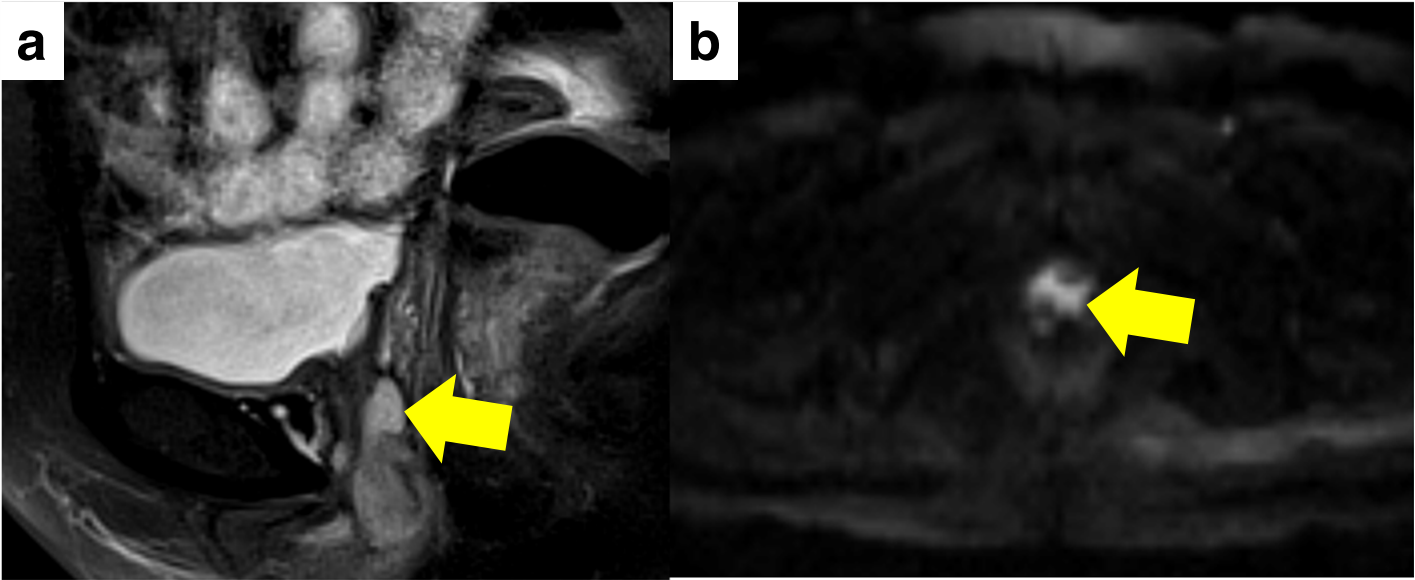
Magnetic resonance images of the urethral tumor (arrows) using (a) T2-weighted imaging and (b) diffusion-weighted imaging
